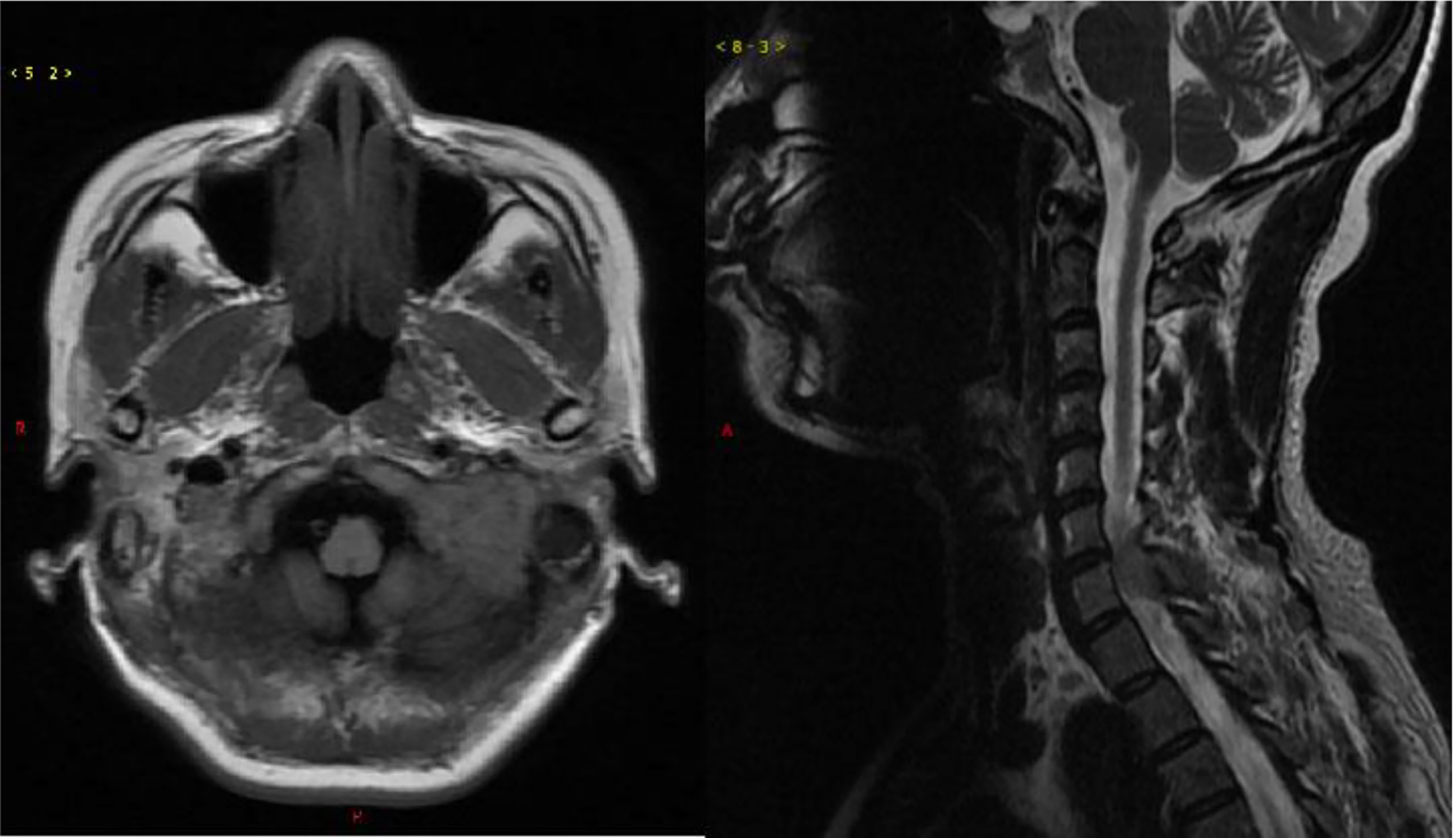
Magnetic resonance imaging of the brain and spine. Left, axial T1 weighted; right, sagittal T2 weighted.
